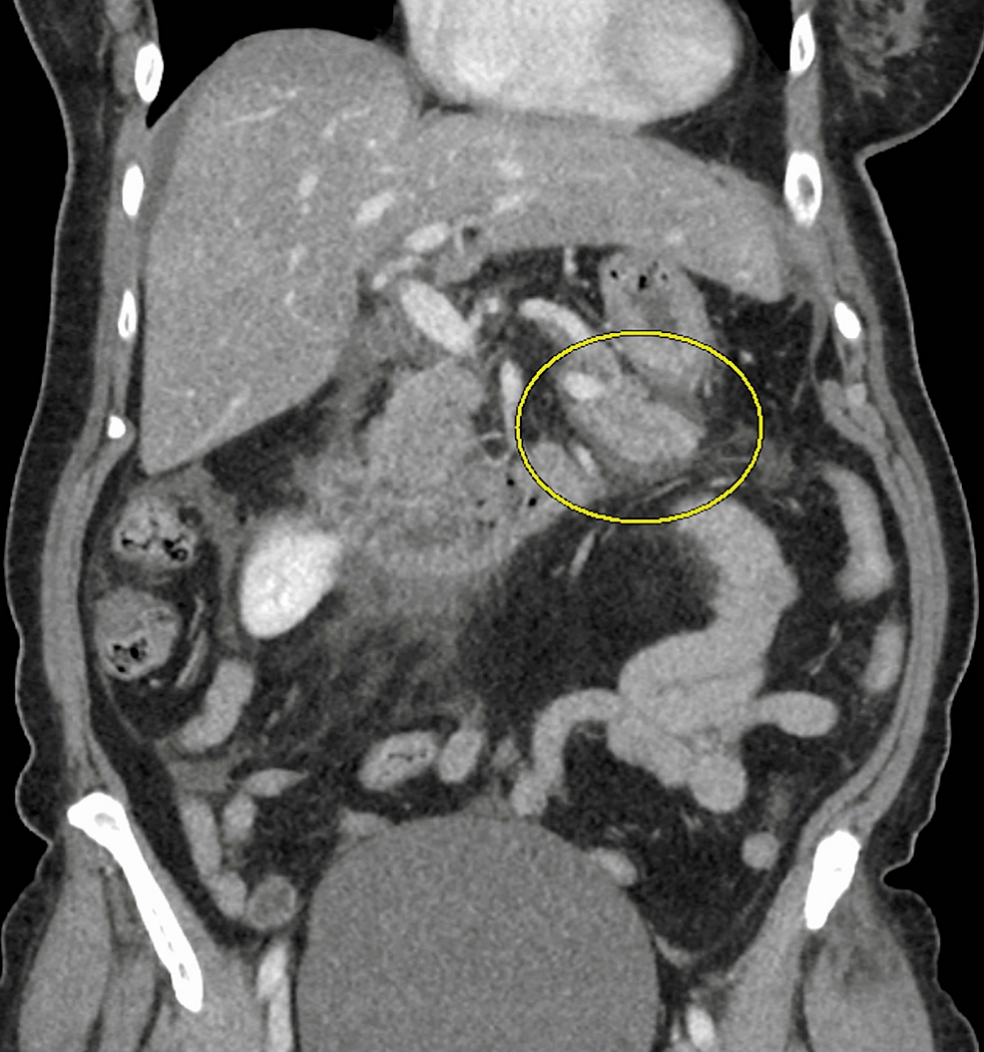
Admission CT suspects pancreatitis. The image is showing fat stranding on the tail of the pancreas (circled).
